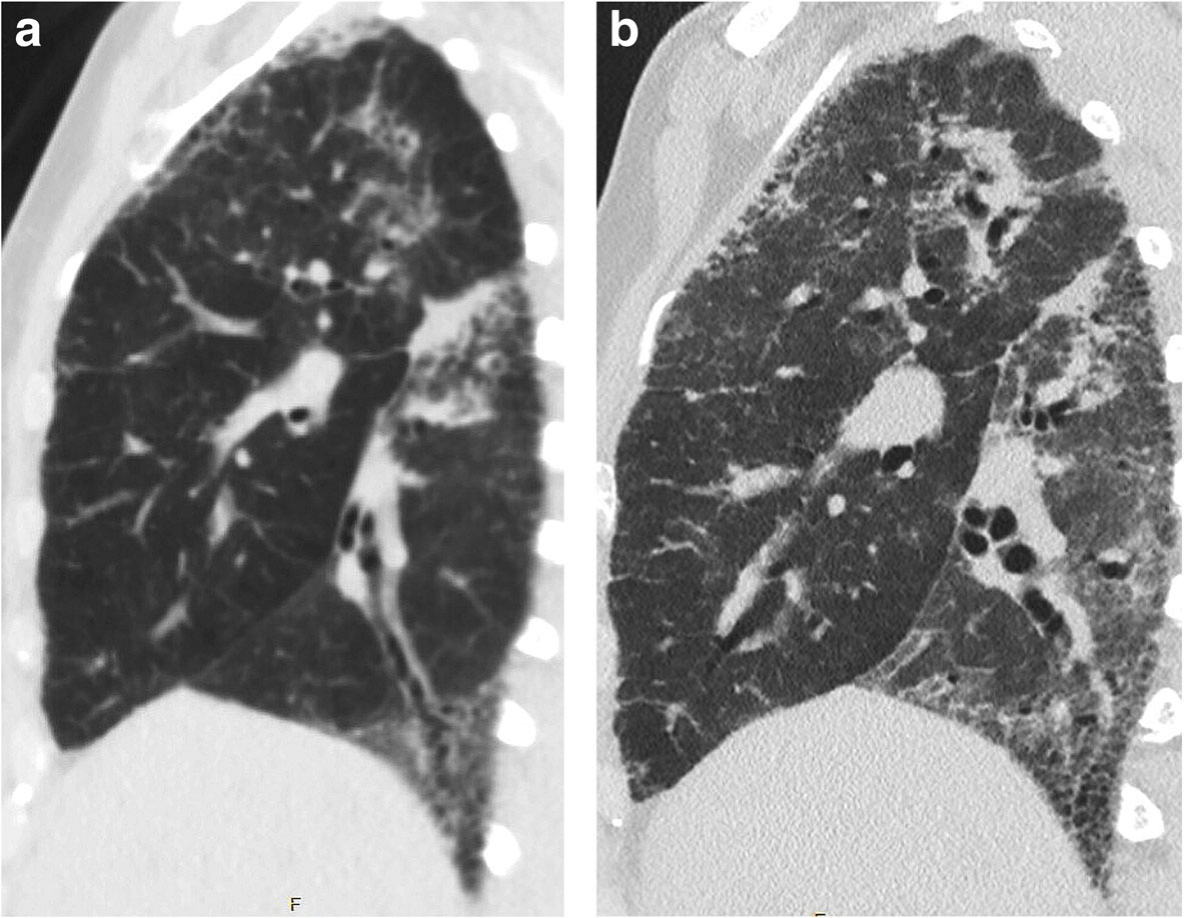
High-resolution computed tomography (sagital plane) of the chest showing worsening of lung fibrosis with thickening of peribronchovascular interstitium, bronchiectasis, reticulations, honeycombing, ground glass opacities and lung parenchyma consolidations. Initial examination (a) and three-month follow-up (b)
